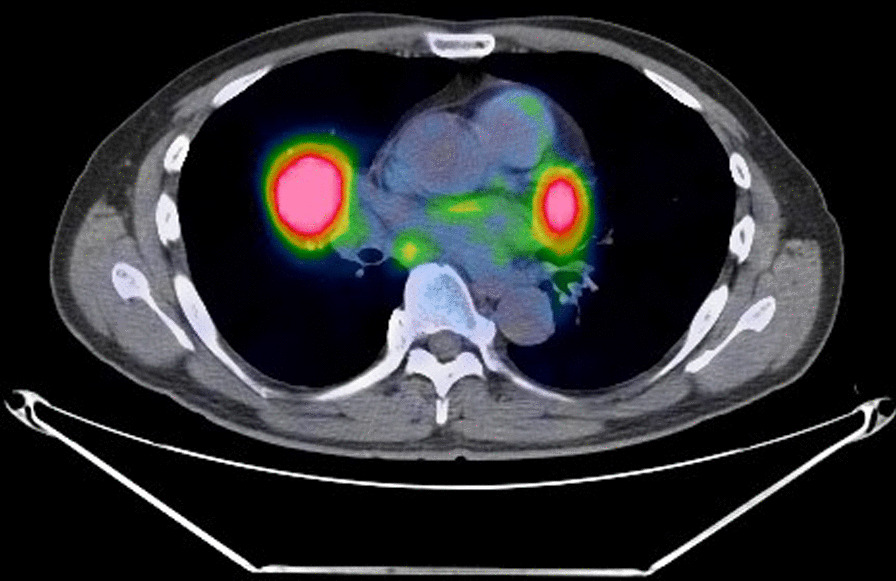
FDG-PET/CT. FDG-PET/CT revealed a mass lesion with a maximum diameter of 5.5 cm, SUVmax of 11.0, and high SUV areas in the hilar and mediastinal lymph nodes
